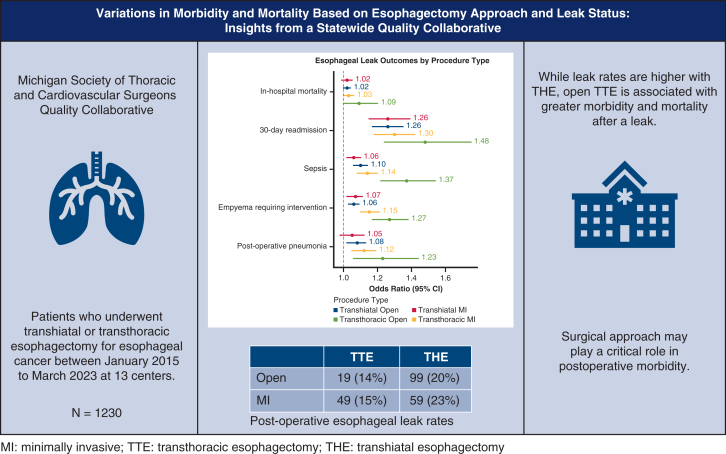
Graphical Abstract- Eight year data from a regional quality collaborative suggests higher leak rates after transhiatal esophagectomy, and higher morbidity and mortality after an open transthoracic esophagectomy.
